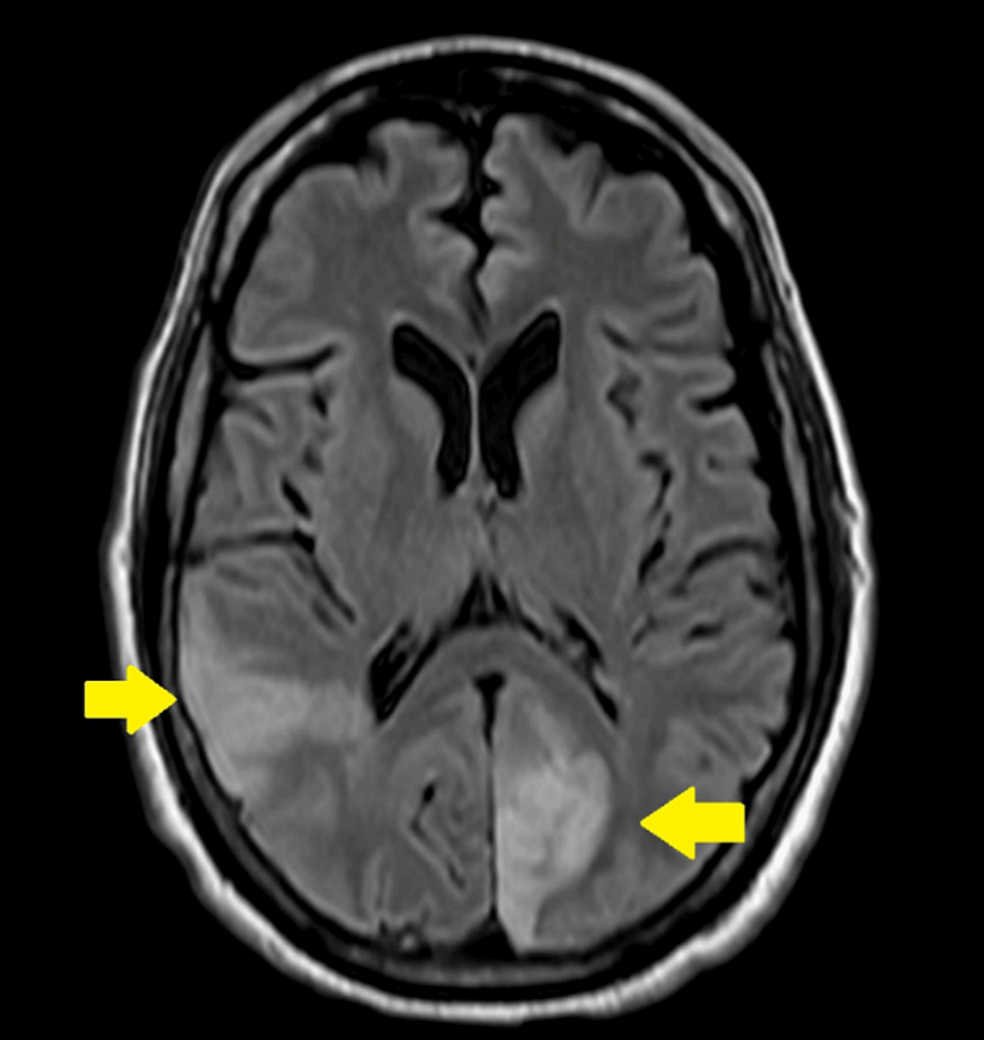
MRI brain showing acute infarcts involving the left medial occipital lobe and the right temporal occipital lobe
